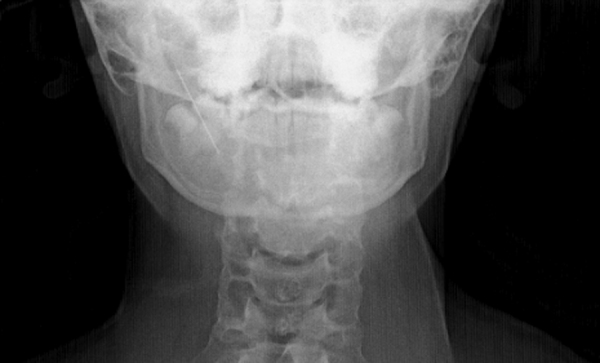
Anteroposterior cervical X-ray.
